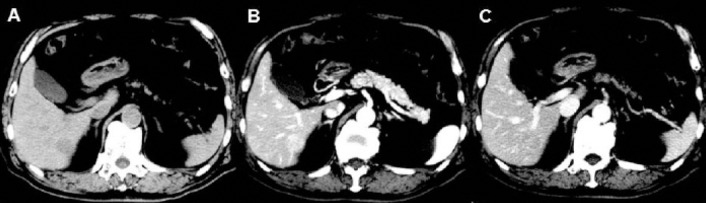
A non-contrast CT image shows a 23×18 mm, well-circumscribed, low-density mass in segment 6 of the liver (A). Dynamic CT images demonstrate the enhancement of the mass in the arterial phase (B) and early washout in the late phase (C)
